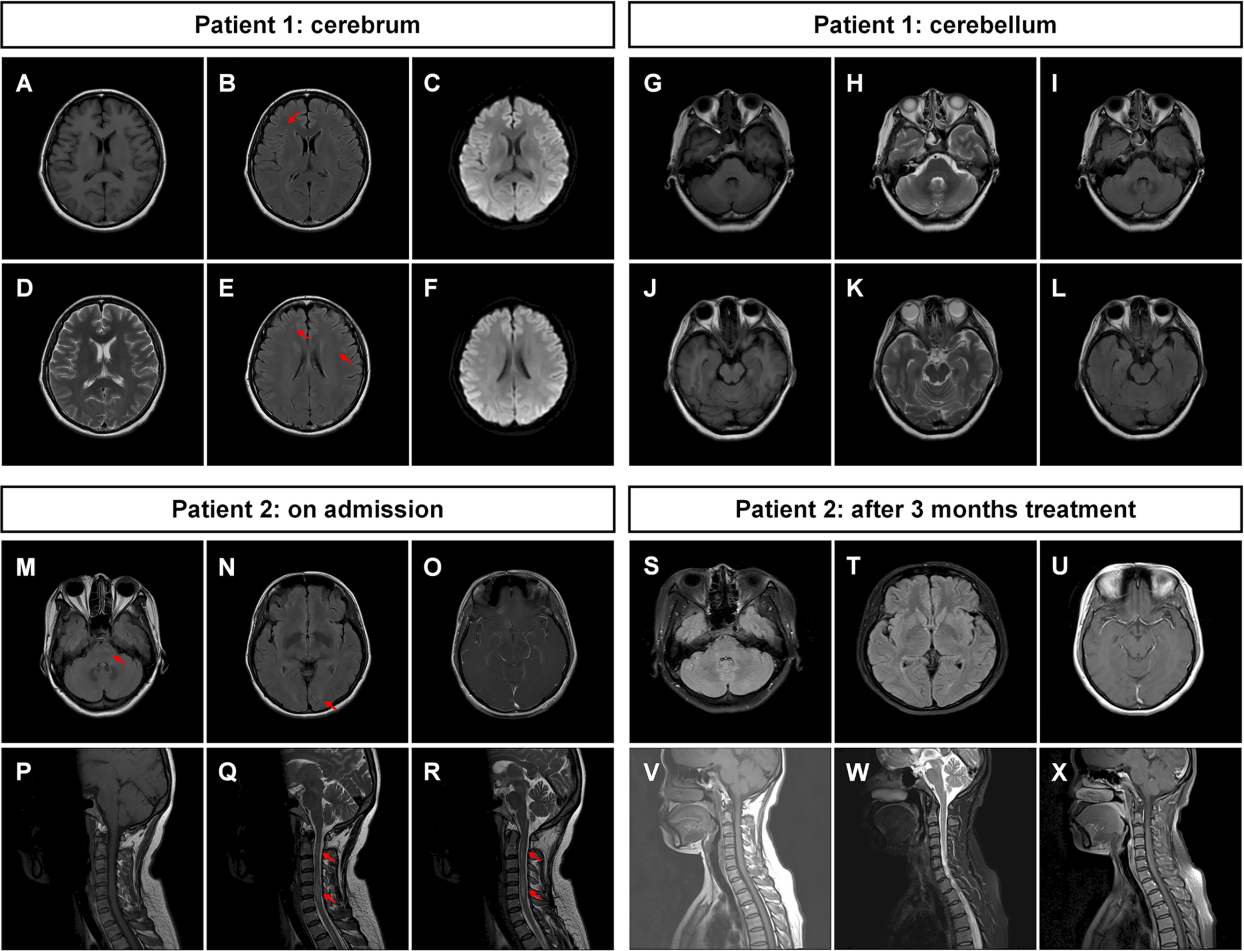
Magnetic resonance images of the patients. (A–F) Brain MRI of patient 1 was not remarkable with mild whiter matter abnormalities (indicated by arrows). (G–L) The MRI of cerebellum was not remarkable. (M–O) Brain MRI of patient 2 showed multiple abnormal signals in white matter in the bilateral cerebral hemisphere and brainstem (indicated by arrows). (P–R) The spine MRI of patient 2 showed long-segment spinal cord lesions from medulla to the C6 segment (indicated by arrows). After 3 months of treatment, (S–U) the brain MRI of patient 2 showed that the abnormal signals in the white matter and brainstem were reduced compared with those in (M–O). (V–X) The cervical MRI showed that the original abnormal signals in the spinal cord had disappeared. T1-weighted images: (A), (G), (J), (P), (V); T2-weighted images: (D), (H), (K), (Q), (R), (W); T2 fluid-attenuated inversion recovery sequence: (B), (E), (I), (L), (M), (N), (S), (T); T1-weighted images with contrast: (O), (U), (X); diffusion-weighted imaging sequence: (C), (F).
